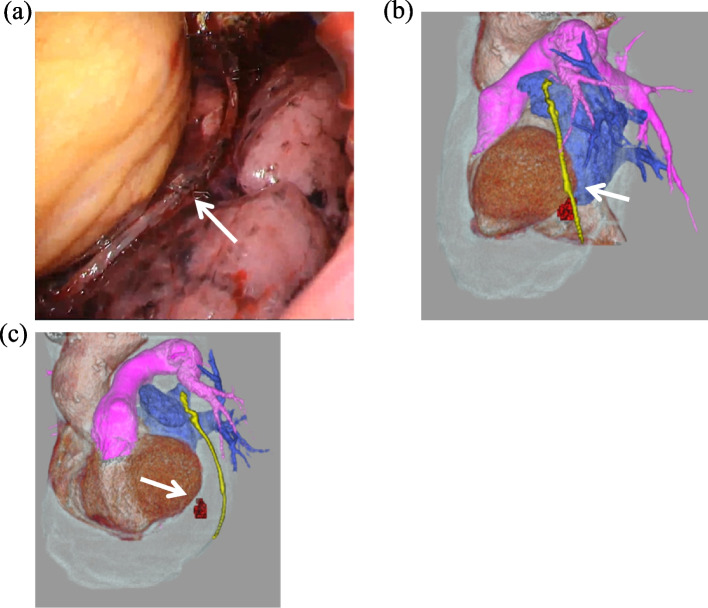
a Intraoperative image of the staple-line that divided the anterior interlober fissure. An intraoperative image of the staple-line that divide the anterior interlober fissure is shown. Although some staples (white arrow) protrude perpendicularly to the cut surface, no malformed staples are noted. b Constructed three-dimensional computed tomography. A three-dimensional computed tomography image is constructed using postoperative enhanced computed tomography. The contrast extravasation (shown in red) is on the staple line dividing the interlobar fissure (shown in yellow). The multiple firing junction is indicated by the white arrow. c Side image of constructed three-dimensional computed tomography. A side image of the constructed three-dimensional computed tomography is shown. This image reveals that the top edge of contrast extravasation is the bleeding point (white arrow), which is close to the multiple firing junction
